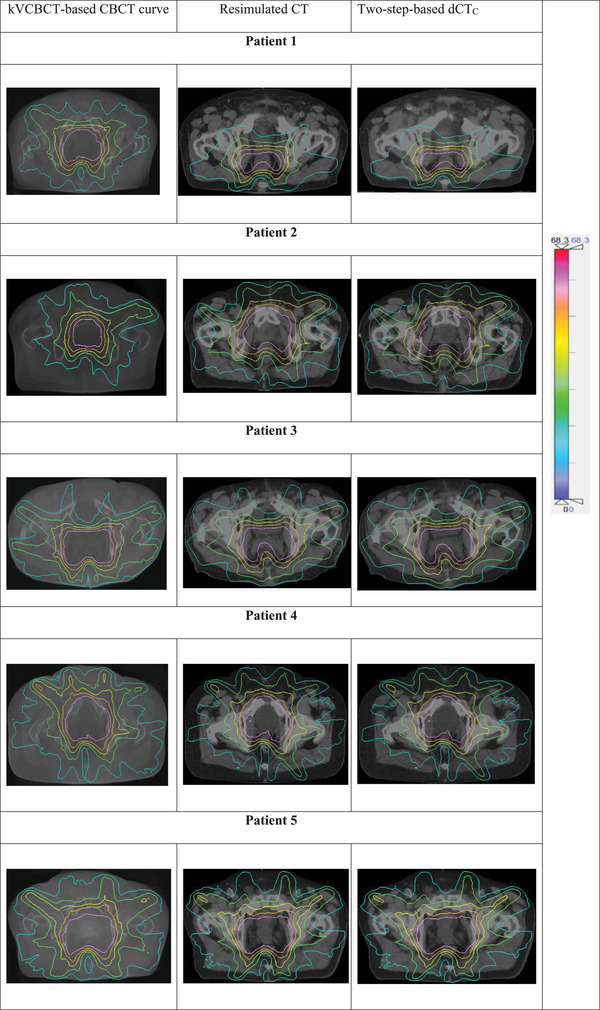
Dose distribution comparison of the kVCBCT, dCT, and rCT images of the five test patients.
